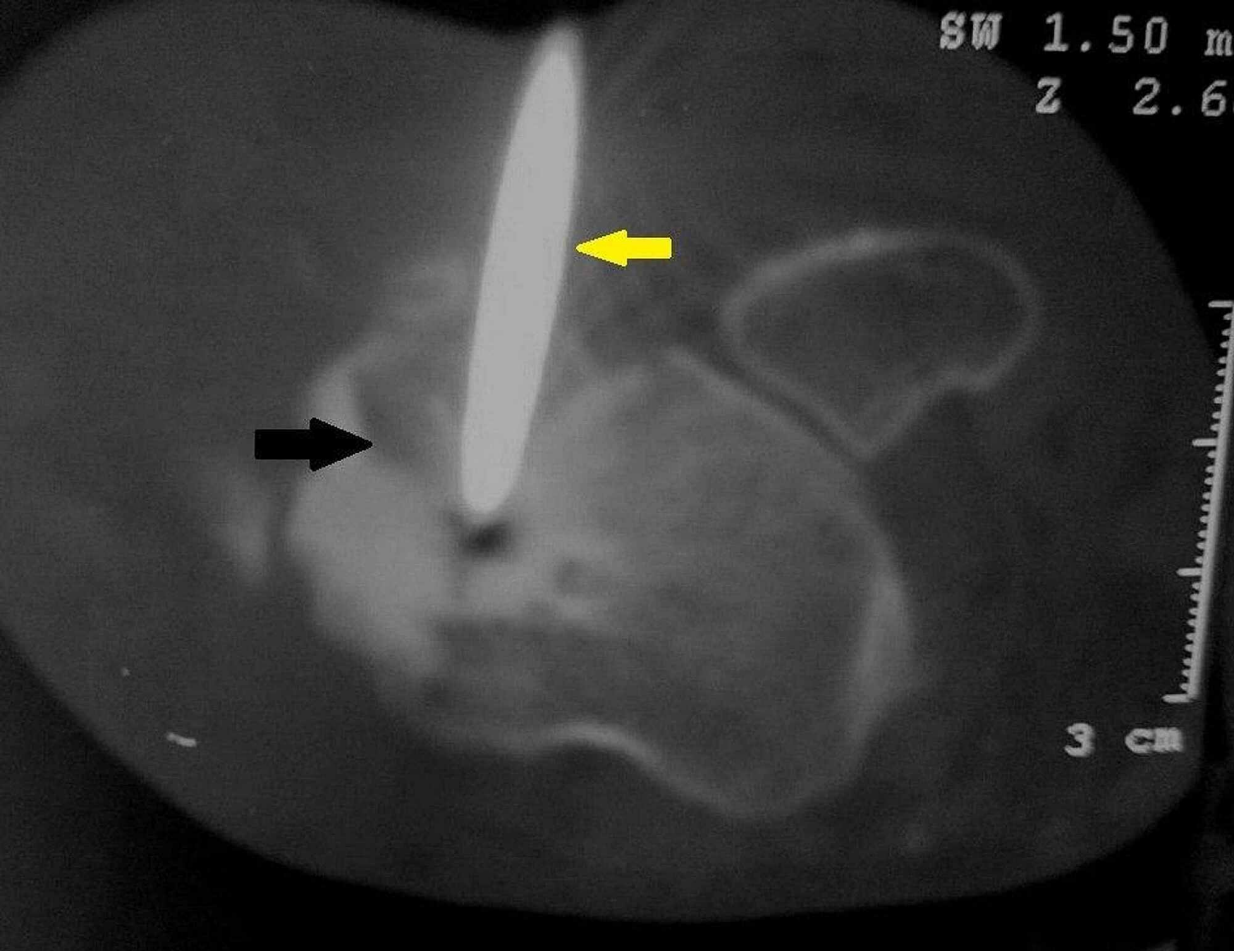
Axial CT of the hindfoot demonstrates CT guided biopsy of the talus neck lesion (black arrow) by the 11G bone biopsy needle (yellow arrow) through anterolateral approach
